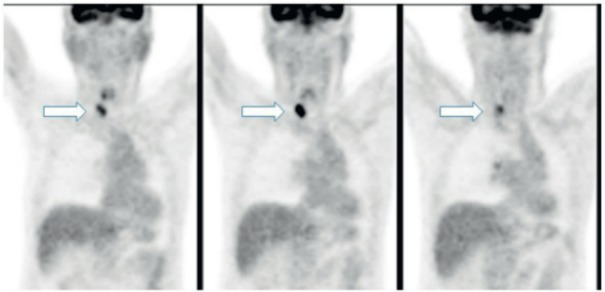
PET-CT: marked uptake in the right lobe of the thyroid gland.
